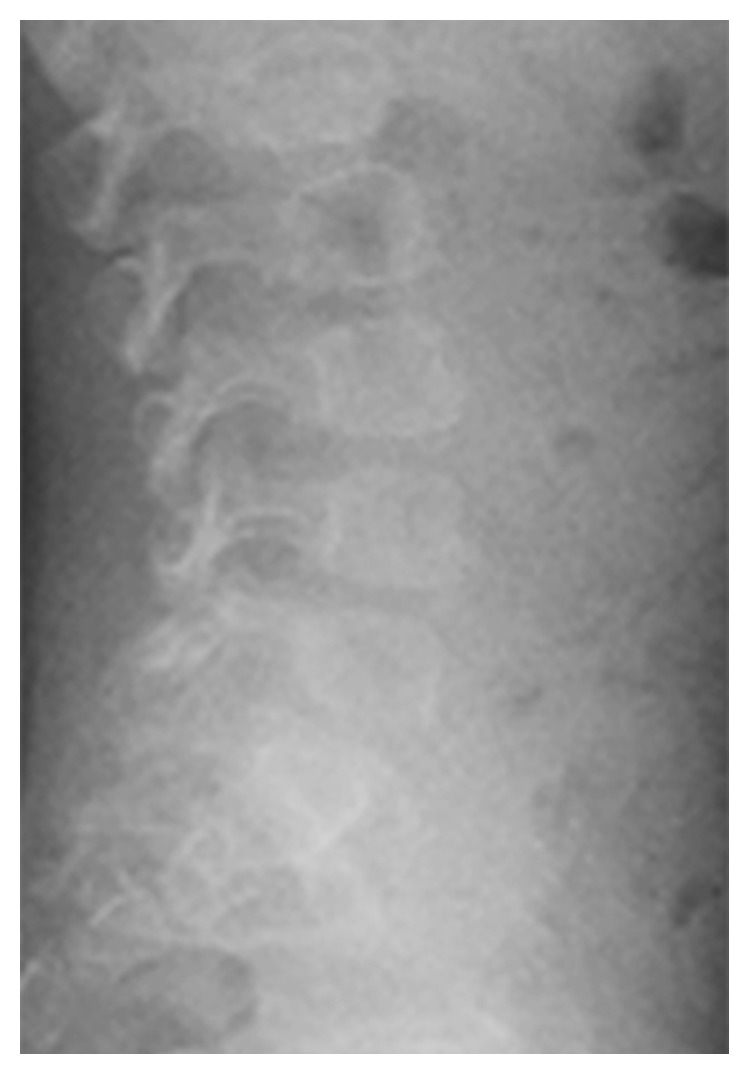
Chest X-ray image revealing egg-shaped vertebra.
